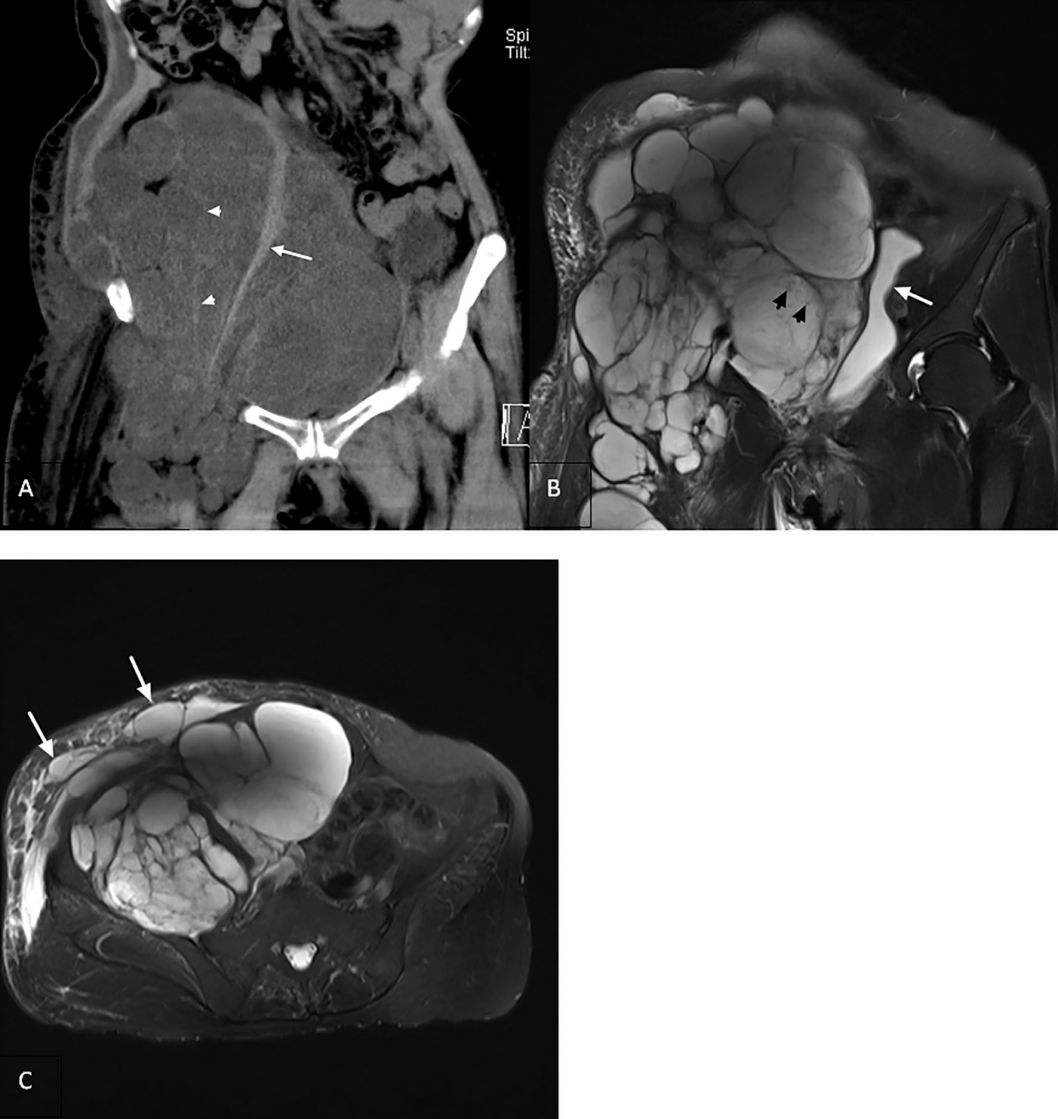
(A) Coronal computed tomography reconstruction (2018) showing a multilocular cystic solid heterogeneous mass in the retroperitoneum with poorly delineated boundaries (arrowhead). Right psoas major muscle showing compressed atrophy and decrease in volume (arrow). (B) Coronal T2-weighted imaging revealing that the lesion invaded the retroperitoneum, inguinal region, and upper right thigh with uneven signal intensity. There are many divergent spots of slightly lower signal intensity (arrowhead) in the lesion, with uneven thickness of the septum. The bladder is compressed and moved to the left (arrow). (C) Axial view showing the right abdominal cyst with lymphedema (arrow).
